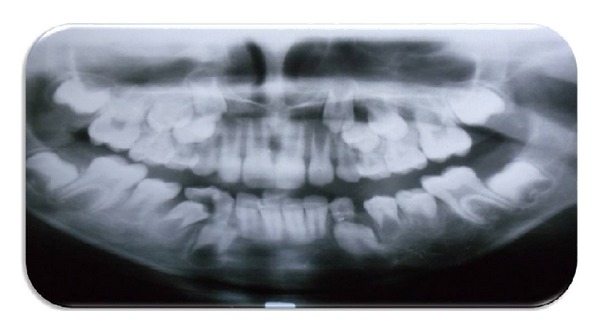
Orthopantomograph showing erupted mandibular left first and second premolars.
